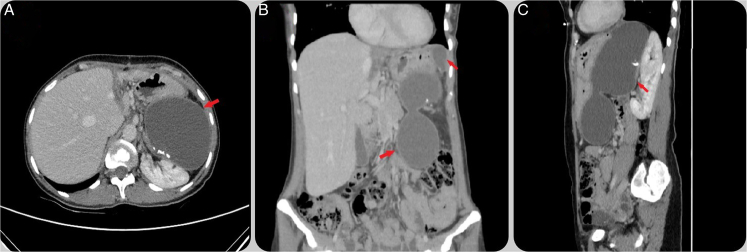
Computed tomography scans of multiple hydatid cysts in the spleen. (A) Axial view of multiple multilocular hydatid cysts (red arrow). (B) Coronal view showing the cysts’ distribution (red arrow). (C): Sagittal view of the cysts’ vertical spread (red arrow).
